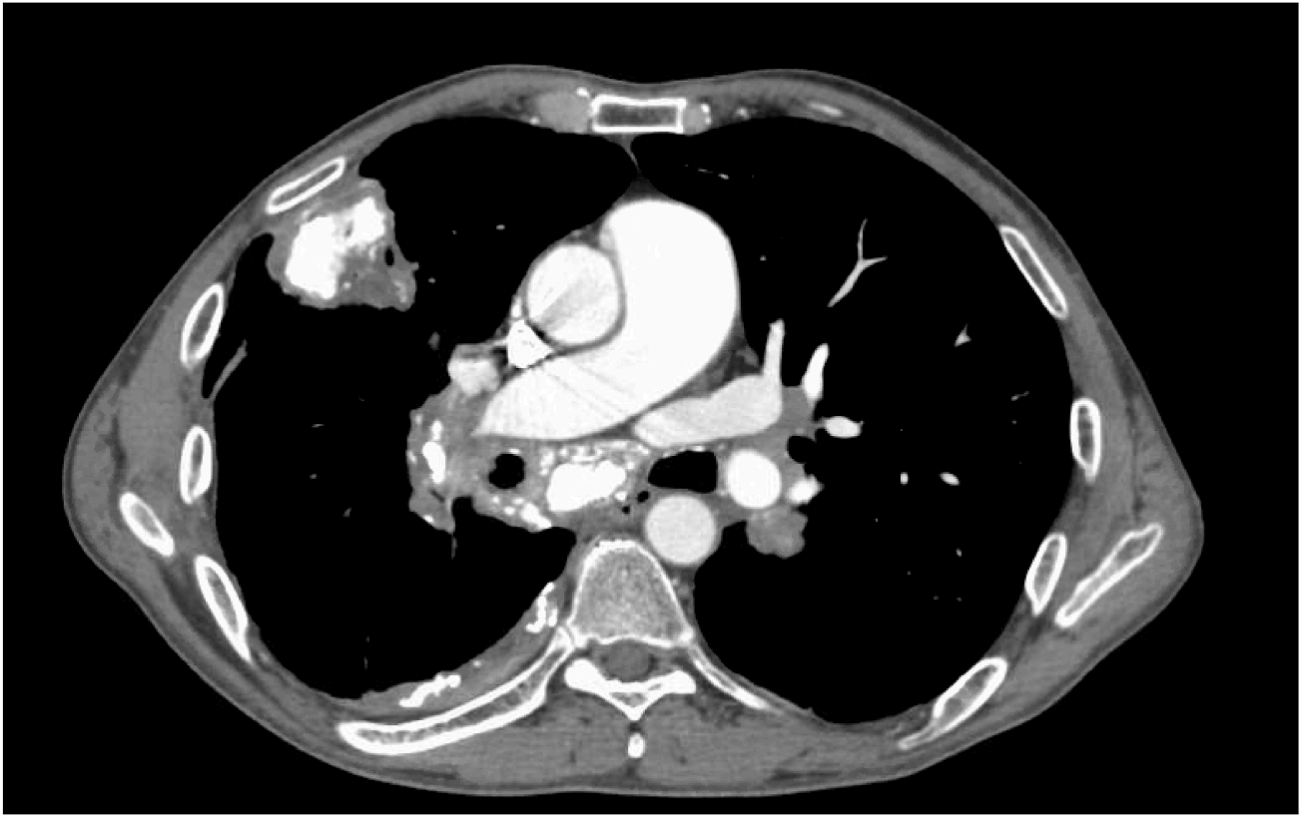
Axial view of computed tomography pulmonary angiography showing severe stenosis of the distal right pulmonary artery. Also in view is calcified mediastinal fibrosis, a calcified pulmonary mass, and a calcified pleural plaque.
